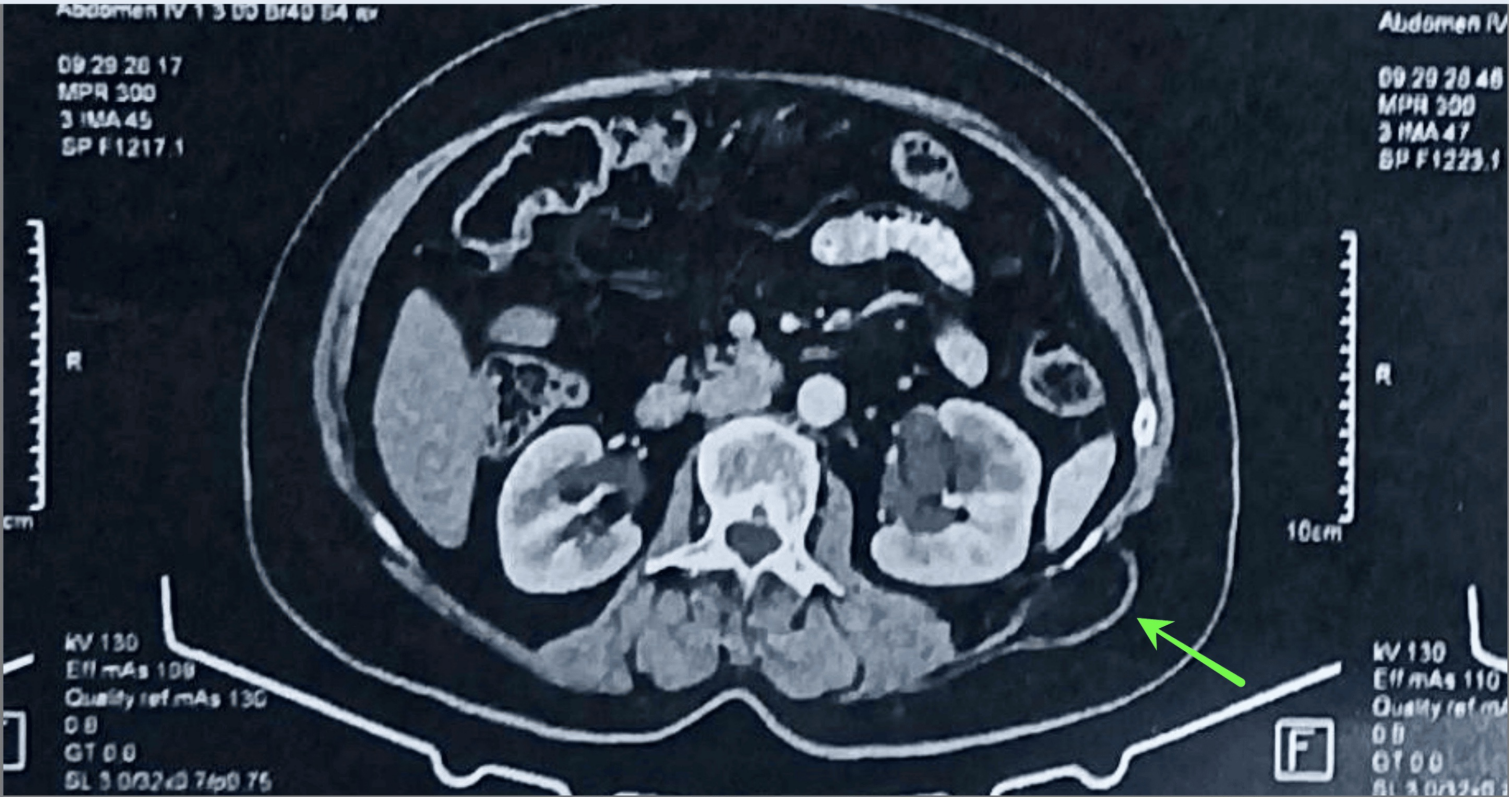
Computed tomography (CT) The CT scan showed a defect in the left lumbar region (green arrow) containing probably extraperitoneal fat. The hernia was suspected to be a Grynfeltt-Lesshaft hernia based on the CT scan and the location of the patient’s pain
